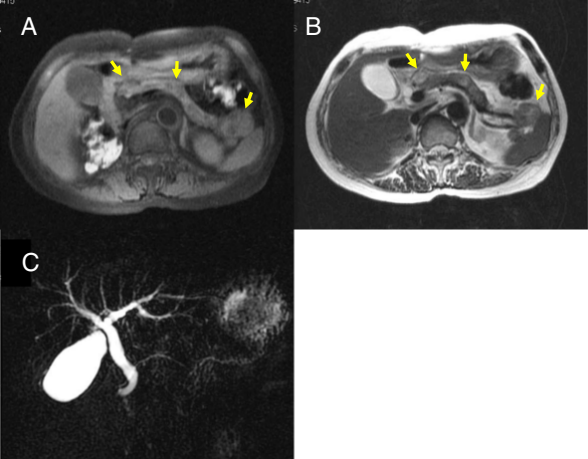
Abdominal magnetic resonance imaging scans (a T1 weighted, b T2 weighted MRI image) showing multiple nodular legions in the pancreas head, tail, and body (yellow arrows). There was no evidence of dilatation of the main pancreatic duct or bile duct (c)
